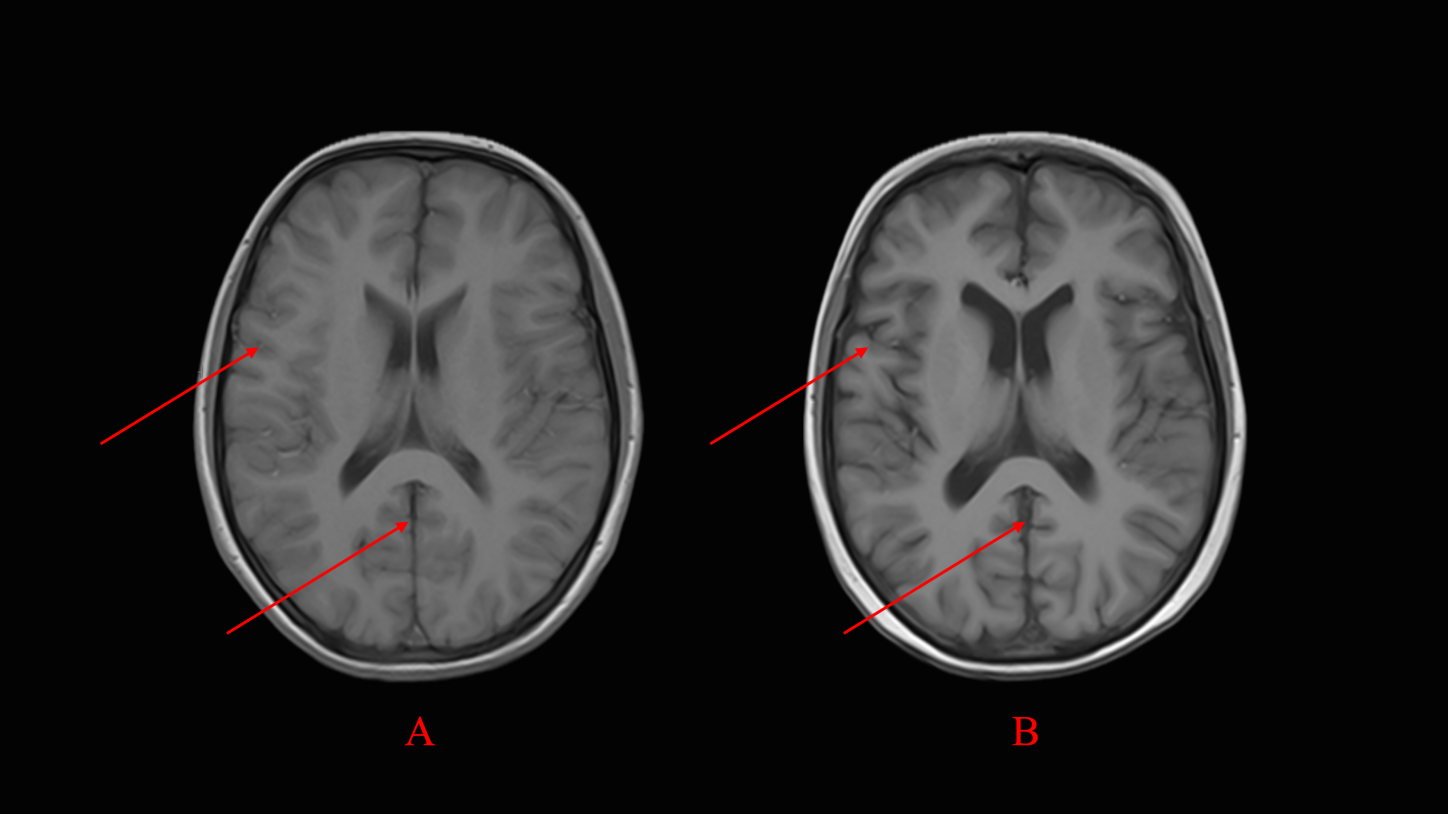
Progressive cerebral atrophy on follow-up MR image. (A) Baseline brain MR image showing mild sulcal widening
